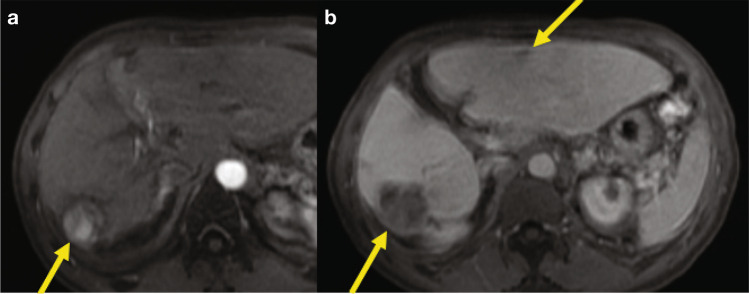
Follow-up post nivolumab therapy demonstrates partial response. Gadoxetic acid-enhanced T1-weighted images during the arterial (a) and PVP (b) demonstrate partial response with decreased size of the arterially hyperenhancing tumor in right hepatic lobe (right arrow) and near-complete resolution of left hepatic lobe tumor (left arrow). *Abbreviation: PVP*, portal venous phase
